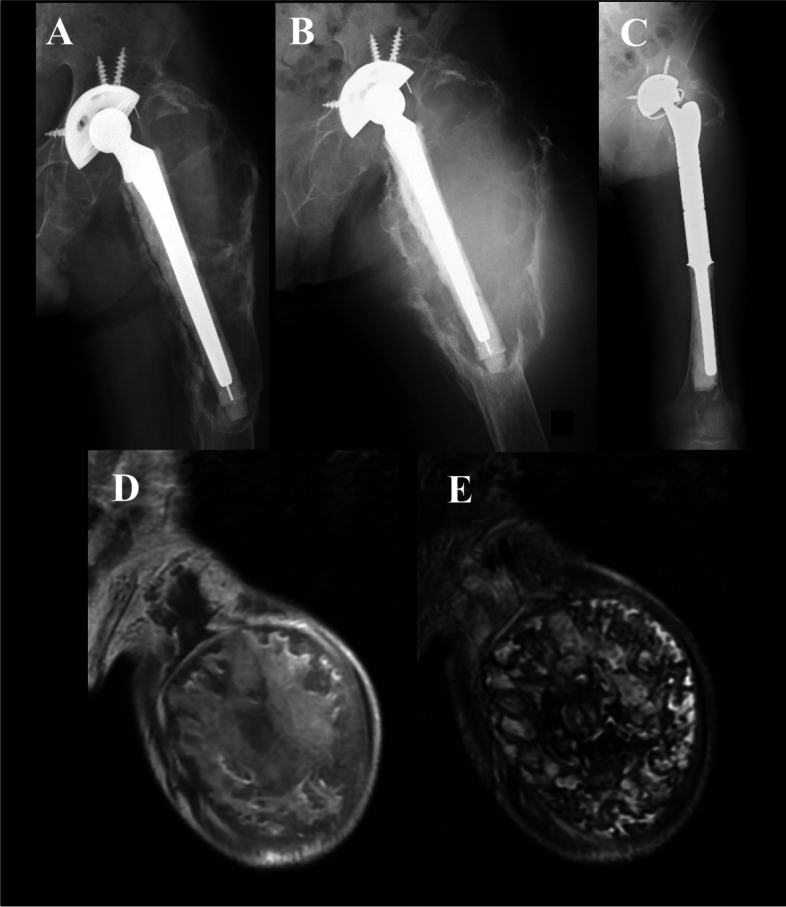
(A) Initial radiograph taken at the first presentation reveals osteolysis, marginal sclerosis, and calcification in the femur, as well as the loosening of the femoral component. (B) Preoperative radiograph taken 2 years after the first visit reveals progressive osteolysis, marginal sclerosis, and calcification in the left femur. (C) No recurrence of intraosseous chronic expanding hematoma is observed at the most recent follow-up, i.e., 6 months postoperatively. The mass predominantly shows a low-signal intensity on a T1-weighted magnetic resonance image (D) and a mixture of low- and high-signal intensities (“mosaic sign”) on a T2-weighted magnetic resonance image (E)
